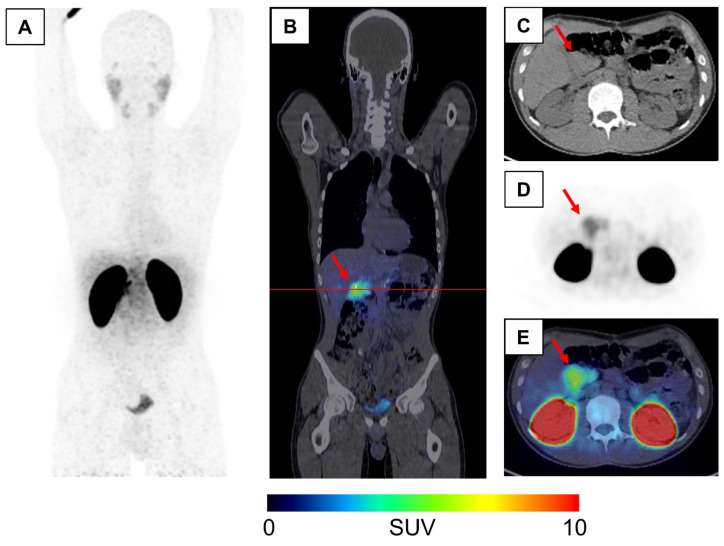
^68^Ga-Exendin PET/CT post left pancreatic resection: Intense tracer accumulation in the remaining pancreatic head led to the decision to complete the pancreatectomy via the Whipple procedure. (A) Maximum intensity projection (MIP) of ^68^Ga-Exendin-PET, (B) Coronar-fused ^68^Ga-Exendin-PET/CT image, (C–E) Axial CT (C), PET (D) and fused (E) ^68^Ga-Exendin-PET/CT images at the height of the pancreatic head (indicated by red line). The red arrow indicates the remaining PET-positive pancreatic head. PET/CT images were windowed using the following parameters: CT: center 50 HU, width 400 HU; PET: 0 (black)—10 (intense red) standardized uptake value (SUV).
